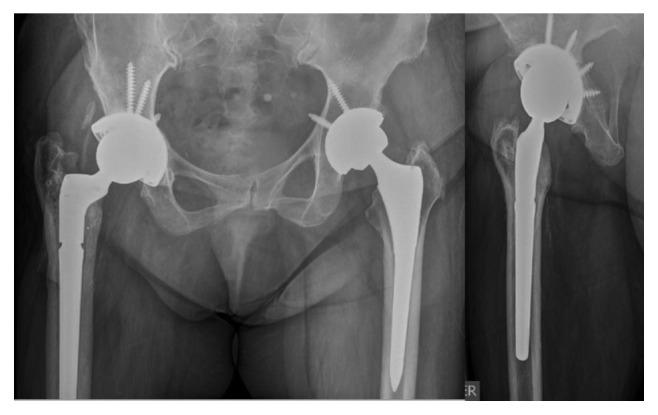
Radiograph at March 2017, 32 months after last surgery. No signs of loosening. Functional scores at final follow-up were HSS: 77.9 points, HOOS: 88.8%, and WOMAC: 93.8%.
